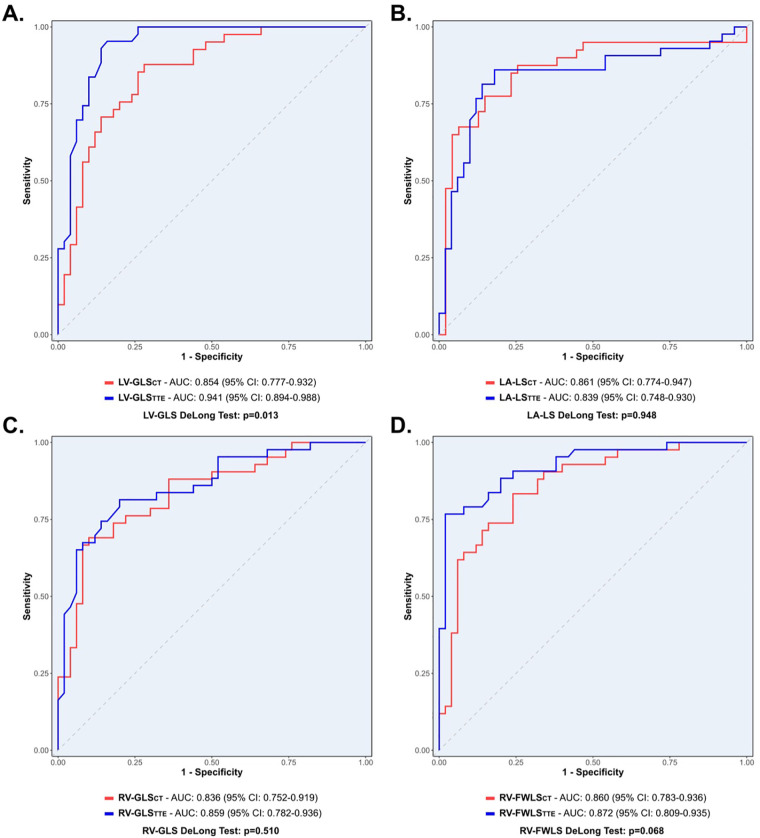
Receiver operating characteristic analyses for detecting H-PH using LV-GLS (A), LA-LS (B), RV-GLS (C), and RV-FWLS (D) from 2D TTE (blue) and 3D CT (red). Receiver operating characteristic curve analyses are reported as the AUC with the corresponding 95% CIs and *p*-values. AUC, area under the curve; CI, confidence interval; CT, computed tomography; H-PH, high probability of pulmonary hypertension; LA-LS, left atrial longitudinal reservoir strain; LV-GLS, left ventricular global longitudinal strain; RV-GLS, right ventricular global longitudinal strain; RV-FWLS, right ventricular free-wall longitudinal strain; TTE, transthoracic echocardiography; 2D, two-dimensional; 3D, three-dimensional.
